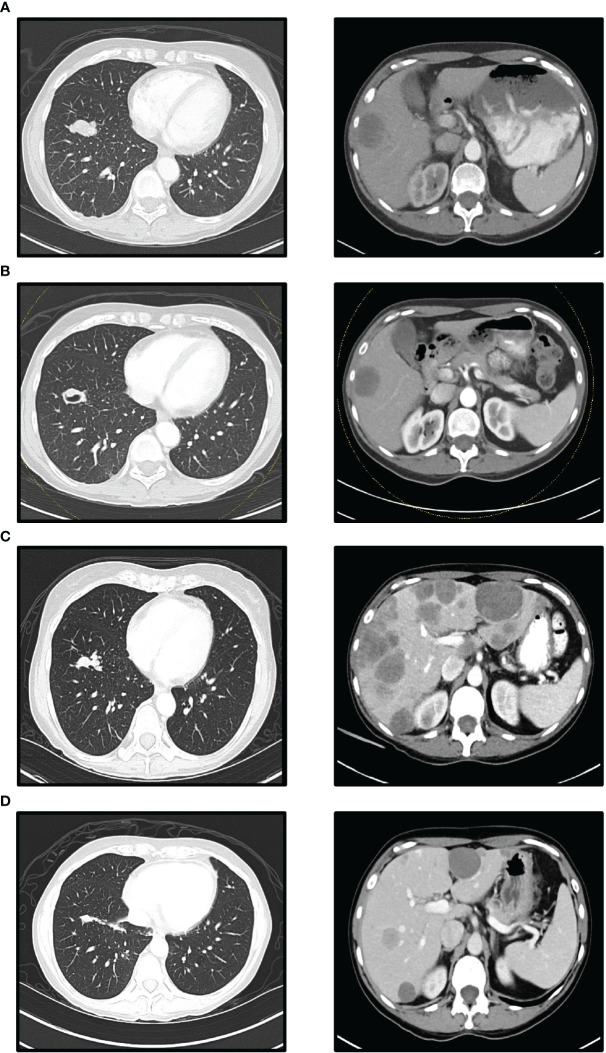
Computed Tomographic (CT) scans (left lower lobe mass and liver metastases): (A) Before nivolumab/ipilimumab treatment. (B) After 42 days of nivolumab/ipilimumab treatment. (C) Before temsirolimus add-on. (D) After 90 days temsirolimus add-on (A, B) show when nivolumab/ipilimumab treatment resulted in a stable disease response for 7 weeks. (C) shows PD response 4 months after starting nivolumab/ipilimumab treatment. The largest liver lesion in (C) measured 88 mm in diameter at its longest. (D) shows significantly reduced lung and liver metastases 3 months after treatment with temsirolimus and nivolumab/ipilimumab.
